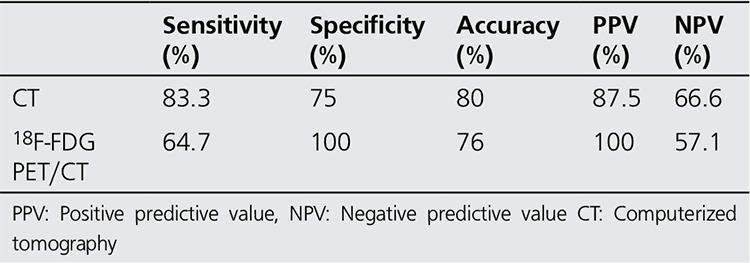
Sensitivity, specificity, accuracy, positive predictive value and negative predictive value of computerized tomography and 18F-FDG PET/CT in the detection of lymph nodes metastasis
